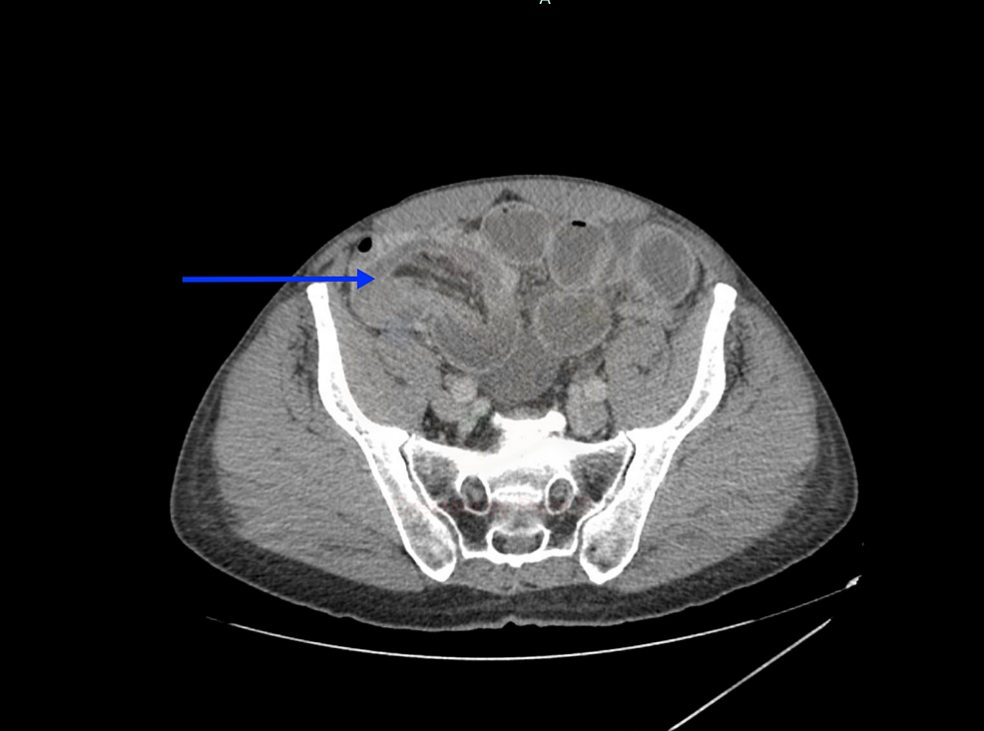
CT abdomen (axial view) showing intussusception of the distal ileum into cecum (blue arrow).
